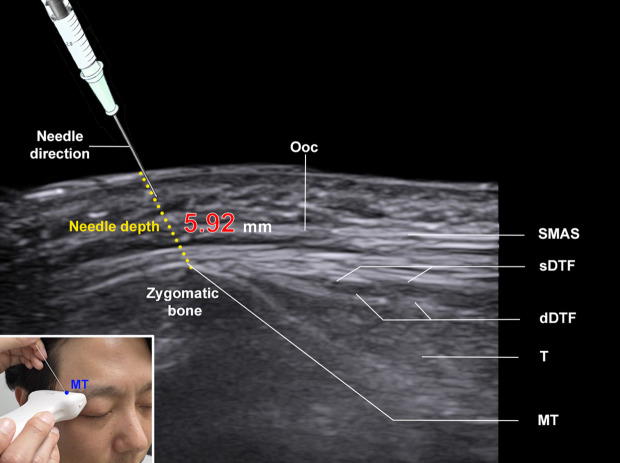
Ultrasonographic image of the temporal region showing the depth from the skin surface to the superficial layer of the deep temporal fascia (sDTF) near the MT. The aZTN was not directly visualized; the measured depth represents an in vivo estimate of the target fascial plane relevant to ZTN/aZTN blockade. Layered structures, including the SMAS, sDTF, deep layer of the deep temporal fascia (dDTF), temporalis muscle (T), orbicularis oculi muscle (Ooc), and zygomatic bone, are labeled. Inset shows probe positioning during in vivo examination.
